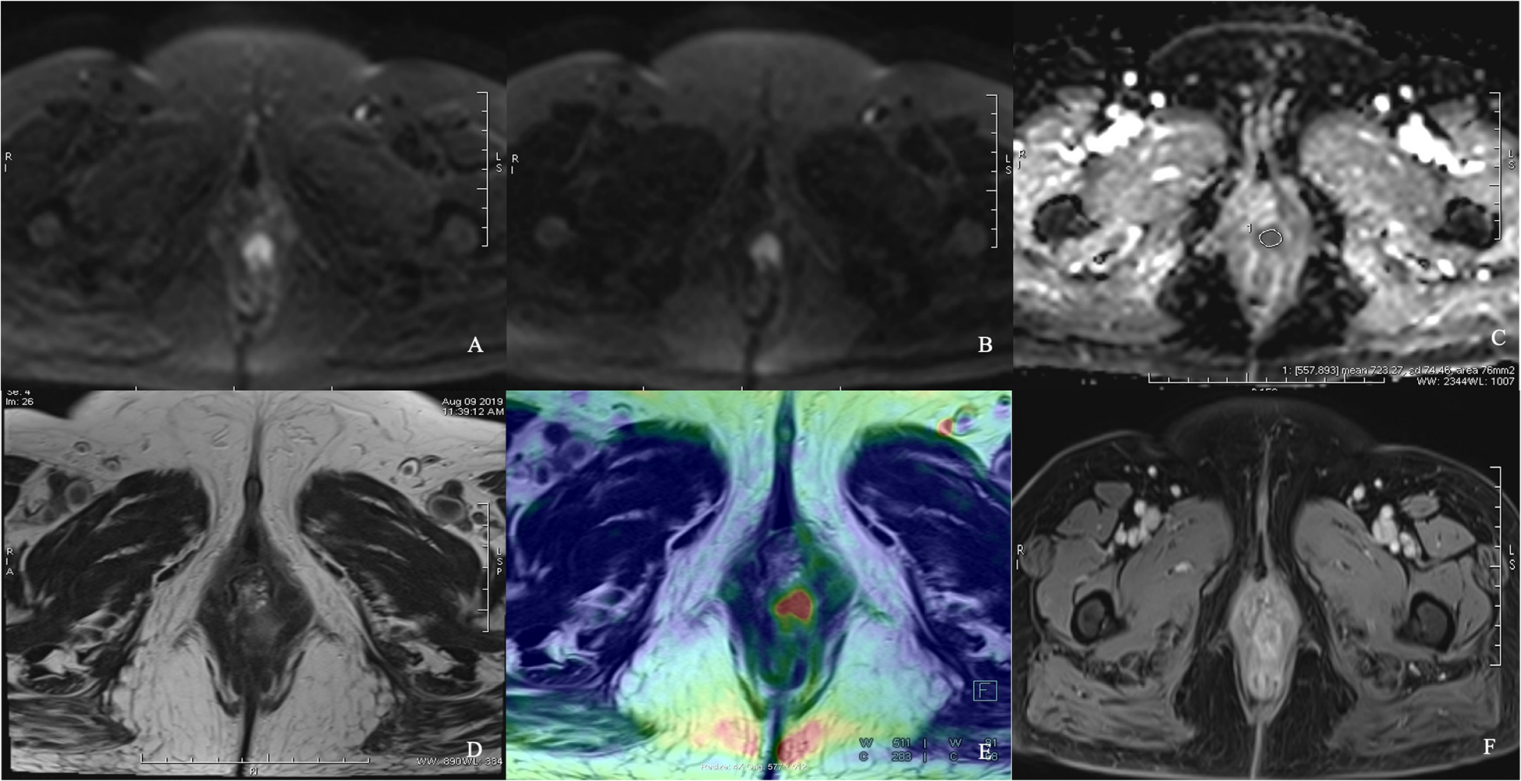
IShim DWI for patient with primary rectal cancer of Grade 3. DW images of b = 800 s/mm^2^ (a), b = 1600 s/mm^2^ (b), ADC map (c), T2WI (d), infusion images of both T2WI and DWI (e) and dynamic T1WI (F) showed the same location of lesion. Average ADC value measured was 723.27 × 10^− 6^ mm^2^/s (c)
